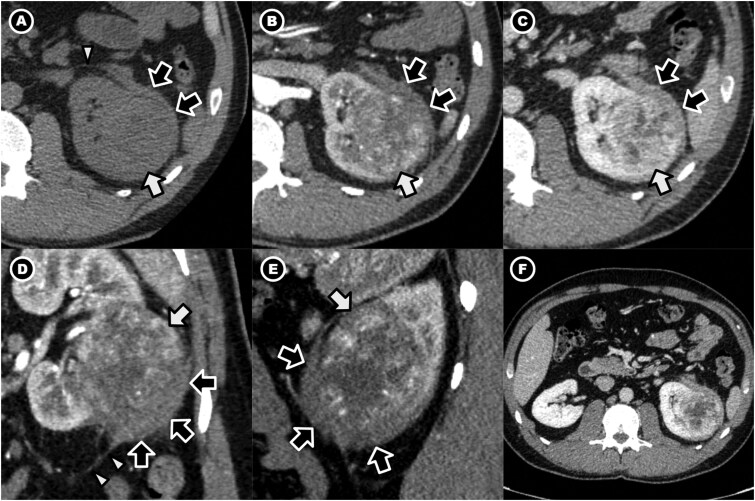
Left renal mass with adjacent perirenal soft tissue. A contrast-enhanced CT scan was performed, including non-contrast, corticomedullary (25-40 s after contrast injection), nephrographic (80-120 s after contrast injection), and excretory (5-10 min after contrast injection) phases. Axial images in the non-contrast (A), corticomedullary (B), and nephrographic (C) phases, as well as coronal (D) and sagittal (E) oblique images demonstrate a heterogeneously enhancing, predominantly endophytic mass (white arrows) in the interpolar region and inferior pole of the left kidney. An adjacent enhancing perirenal soft tissue focus (black arrows) is inseparable from the mass, infiltrates the perirenal fat, and extends beyond Gerota’s fascia (arrowheads). These findings were initially interpreted as perinephric invasion of the renal tumour, consistent with cT4 disease. The excretory phase is not shown, as it was not contributory to the renal mass evaluation. (F) Axial nephrographic phase image at the level of both kidneys demonstrates unilateral involvement confined to the left kidney and perinephric region, with no contralateral or central retroperitoneal abnormality.
